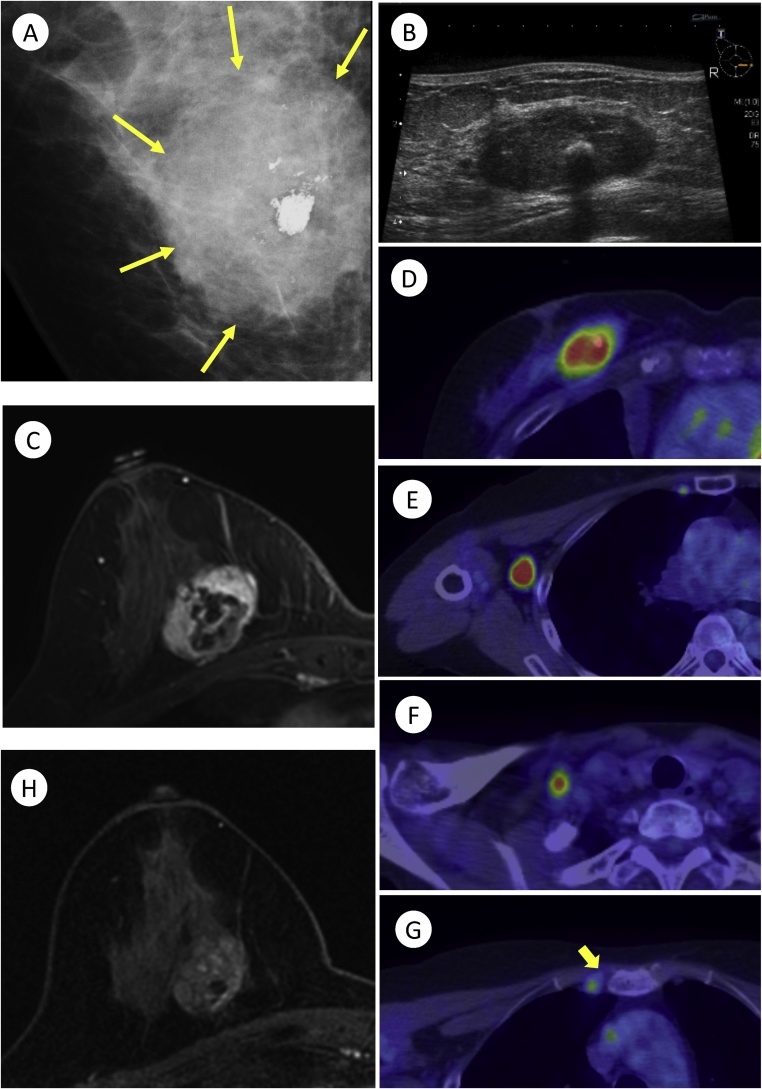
Pre-NAC imaging examinations of the right breast tumor (Fig. 1A–G). A: Mammography showed a solitary mass with ill-defined margins, a coarse calcification, and pleomorphic calcifications (yellow arrow). B: Ultrasonography showed a solitary mass with smooth or irregular margins. C: Dynamic contrast-enhanced T1-weighted 3.0 T magnetic resonance imaging (MRI) with fat suppression (90 s) showed a 3.6-cm oval mass with partially ill-defined and markedly enhanced margins. High FDG uptake for right breast tumor (D), regional lymph node (E), supraclavicular lymph node (F), and internal mammary lymph node (G; yellow arrow) in positron emission tomography-computed tomography (PET-CT) imaging. Post-NAC imaging examinations of the right breast tumor (Fig. 1H). H: 3.0 T MRI (90 s) after receiving neoadjuvant chemotherapy showed the remaining 2.7-cm tumor with a reduction in size and decreased enhancement.
